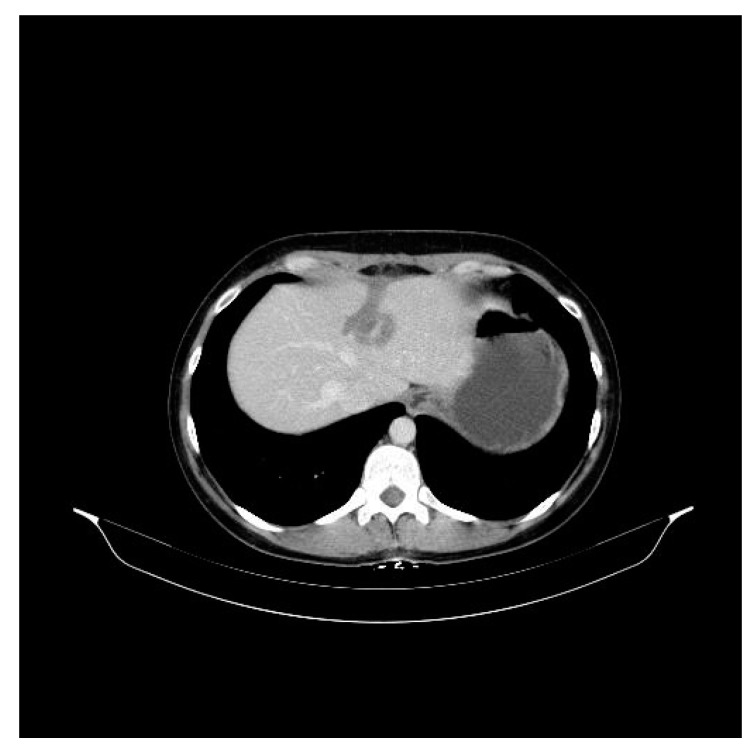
Aspect on the abdominal CT in 2017 after medication with docetaxel 75 mg/m^2^ iv, trastuzumab 600 mg/5 mL sq./21 days and medical ovarian suppression (goserelin 3.6 mg sq./28 days) with the liver metastasis (15 mm diameter).
